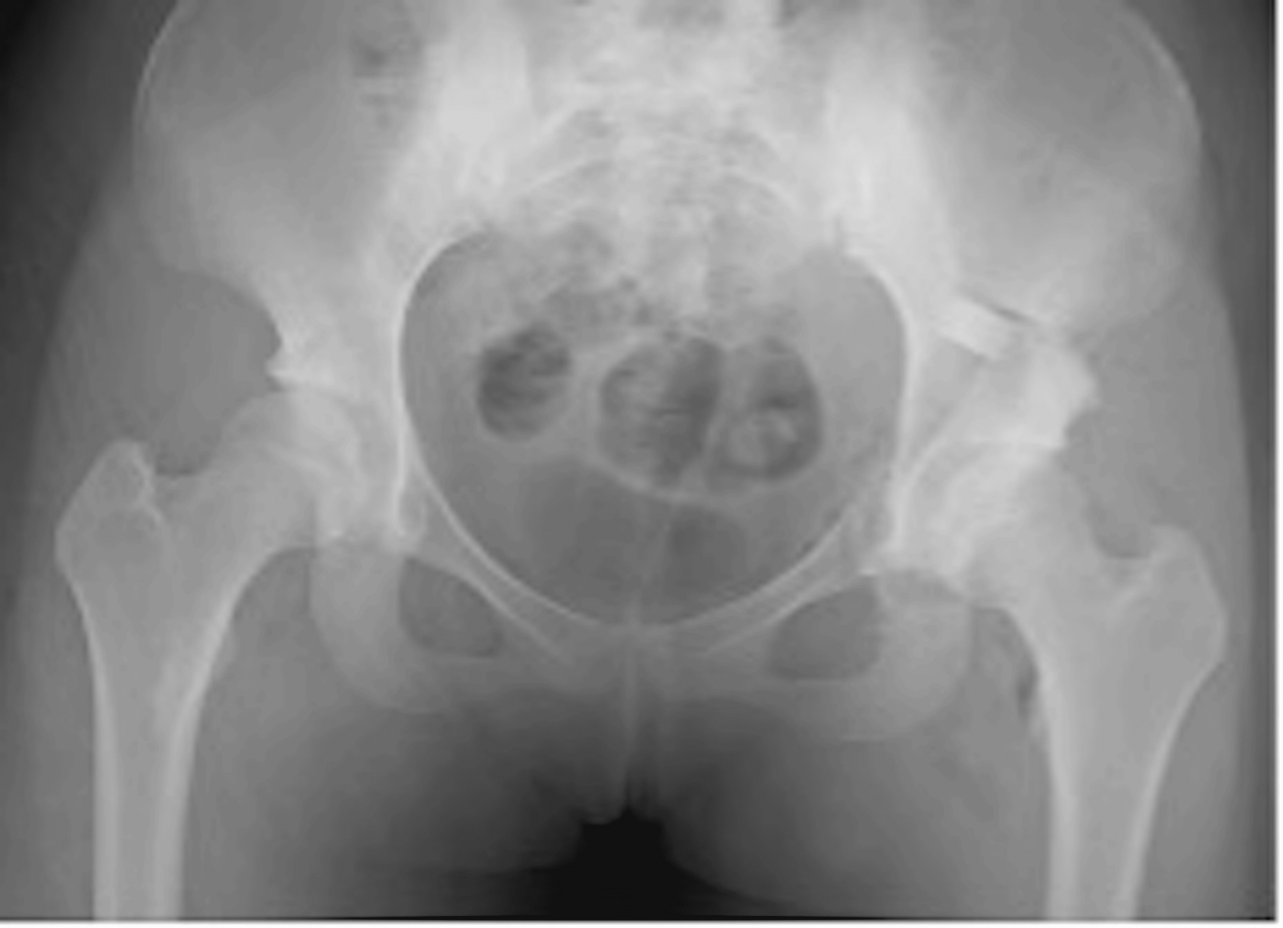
Postoperative radiographs of case 2. CE angle: 25.4°, ARO: 0.3°, Sharp's angle: 51.6°, AHI: 80.0%. CE, center–edge; ARO, acetabular roof obliquity; AHI, acetabular head index
